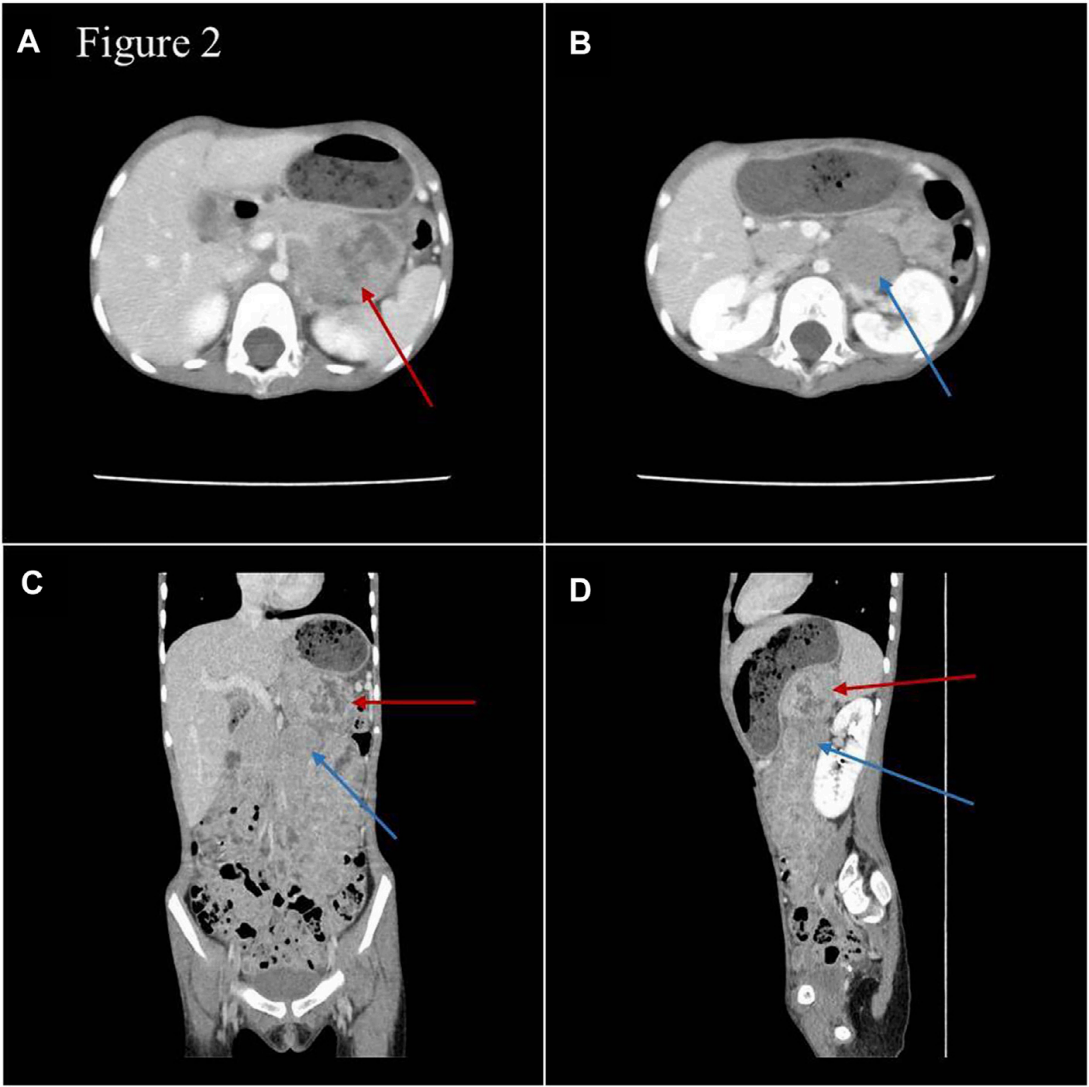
Images of abdominal enhanced CT (A,B) and three-dimensional reconstruction (C,D). Space-occupying lesions with inhomogeneous enhancement were in the body and tail of the pancreas (red arrow) and in front of the left renal vein (blue arrow), and the paraaortic lymph nodes were enlarged.
